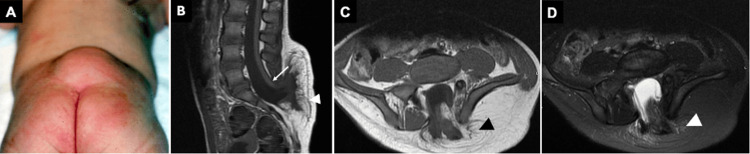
(A) Clinical presentation of lipomyelomeningocele demonstrating a subcutaneous mass above the gluteal crease. Sagittal (B) and axial (C) T1-weighted and STIR (D) MR images of a lipomyelomeningocele show a characteristic low-lying conus (white arrow) posteriorly displaced with concomitant herniation of lipomatous tissue, which is attached to the neural placode (white and black arrowheads) through the defect. STIR, short tau inversion recovery All images were obtained by the authors from the institutional picture archiving and communication system (PACS). They have been de-identified and used in compliance with institutional policies.
